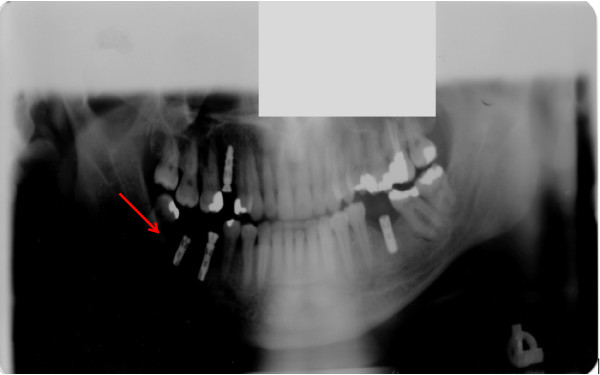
Panoramic radiograph of patient’s jaws. Shows no evidence of intra-bony pathology in association with the soft tissue lesion (arrow).
